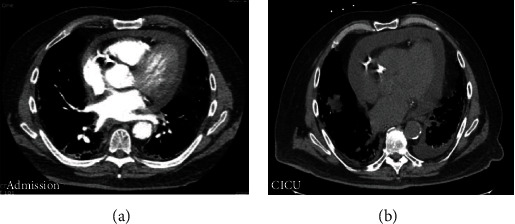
CT chest on hospital day 1 (a) and hospital day 7 (b). The pericardial effusion had expanded from a largest diameter of 1.5 cm (a) to 2.0 cm (b) along with increase in left pleural effusion.
